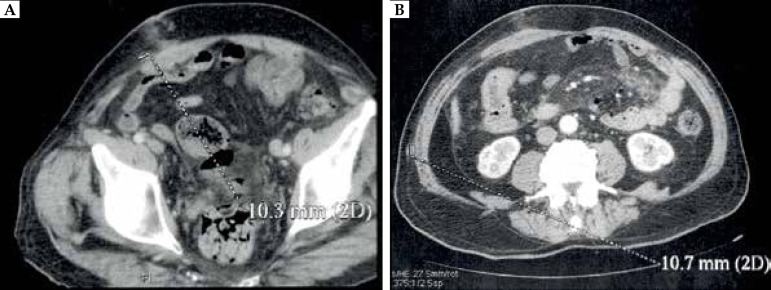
Postoperative abdominal CT. Subcutaneous metastases a) 10.3
